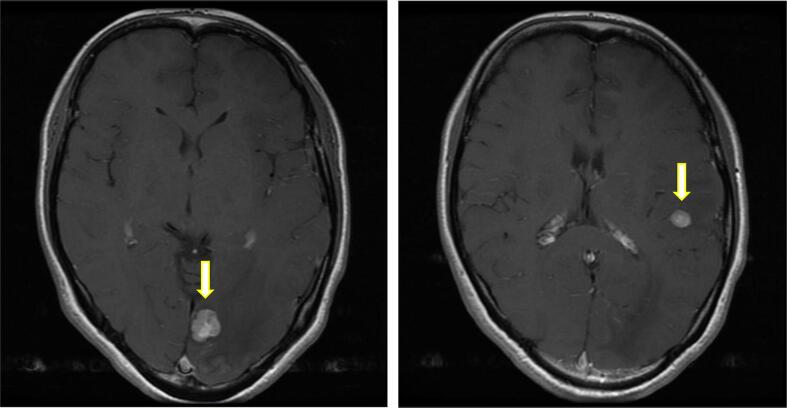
Multiple brain metastases in the left frontal, parietal, and occipital lobes (arrow, left) and the left cerebral hemisphere (arrow, right) in Case 2 (axial post-contrast T1-weighted brain MRI).
